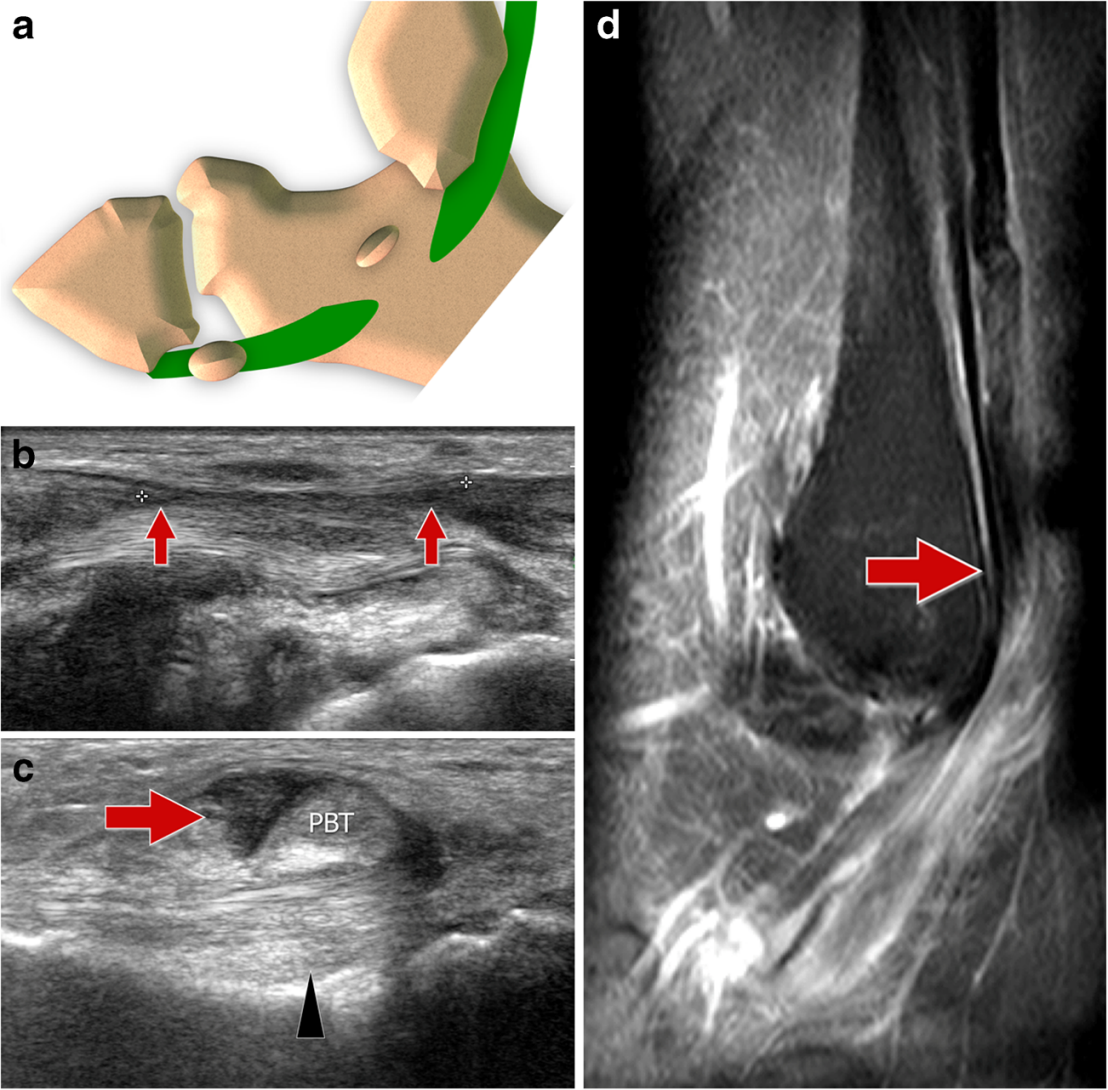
PLT tear type I. *12A* Scheme *12B* Ultrasound image. Longitudinal scan (tendon long axis). *Arrows* show the two tendon stumps and the gap between them. *12C* Ultrasound image. Transverse scan (tendon short axis). The peroneus longus tendon is not visible and is replaced by effusion (*arrow*). Peroneal calcaneal ligament (*arrowhead*). *PBT* peroneus brevis tendon. *12D* Magnetic resonance imaging. Retracted proximal stump of the peroneus longus tendon (*arrow*)
